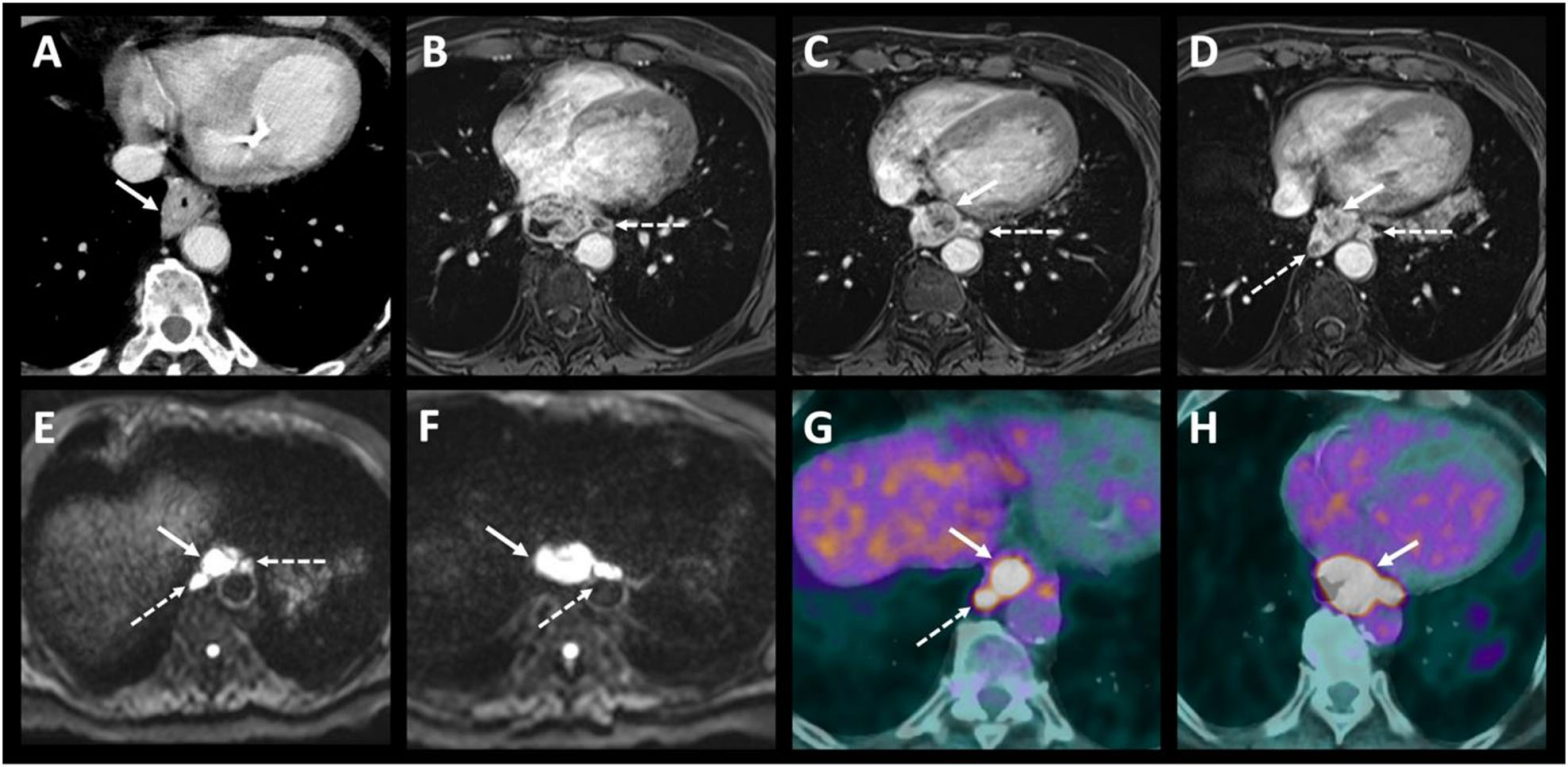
Example 3—MRI and PET/CT for lymph node evaluation. A 70-year-old patient with squamous cell carcinoma located in the lower third of the esophagus. Contrast-enhanced CT image (A) depicted the tumor without lymphadenopathy, while enhanced-T1 MR (B–D) and diffusion-weighted MR (E, F) images show the esophageal tumor (white arrows) but also multiple adjacent lymphadenopathies (white dotted arrows). The primary esophageal tumor (white arrow) and adjacent lymphadenopathies (white dotted arrows) were also clearly depicted on PET/CT images (G, H)
